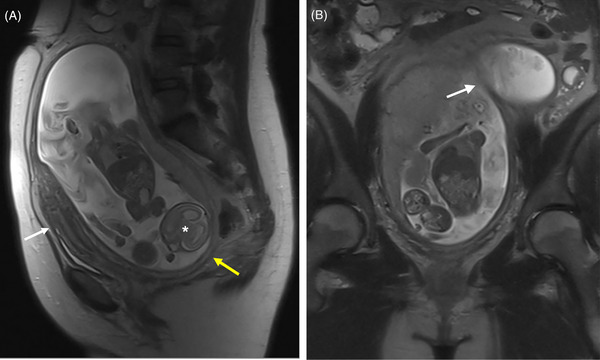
(A) Midline sagittal T2‐weighted MRI of the pelvis demonstrating incarcerated uterus with elongation and anterior displacement of the cervix (thin white arrow). The uterine fundus (thick yellow arrow) and the head of Fetus 2 (asterisk) are located in the presacral space. (B) Coronal T2‐weighted MR image of the pelvis demonstrating a T2 hypointense fibrous band at the uterine fundus (arrow).
